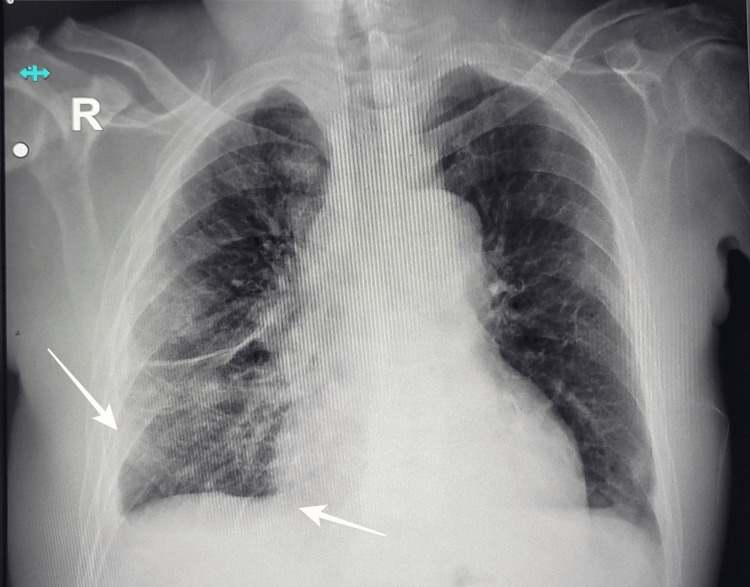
Chest radiograph demonstrating prominent bronchovascular markings (arrows), no other relevant cardiovascular or lung findings.
